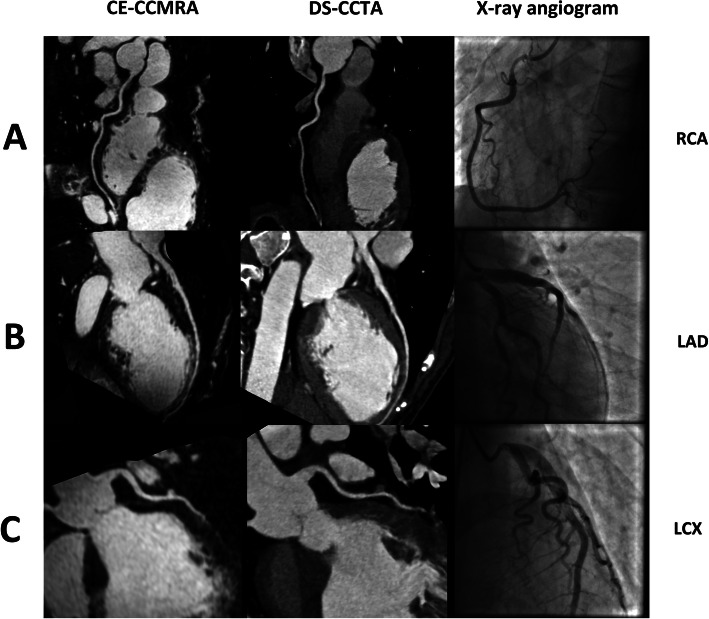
Typical examples of contrast enhanced (CE) coronary cardiovascular magnetic resonance angiography (CCMRA) (left), and dual source (DS) coronary computed tomography angiography (CCTA) (center), and corresponding x-ray angiography images (right) of the right and left coronary artery system. a. Normal right coronary artery (RCA). b. Normal left anterior descending coronary artery (LAD). c. Normal left circumflex coronary artery (LCX)
